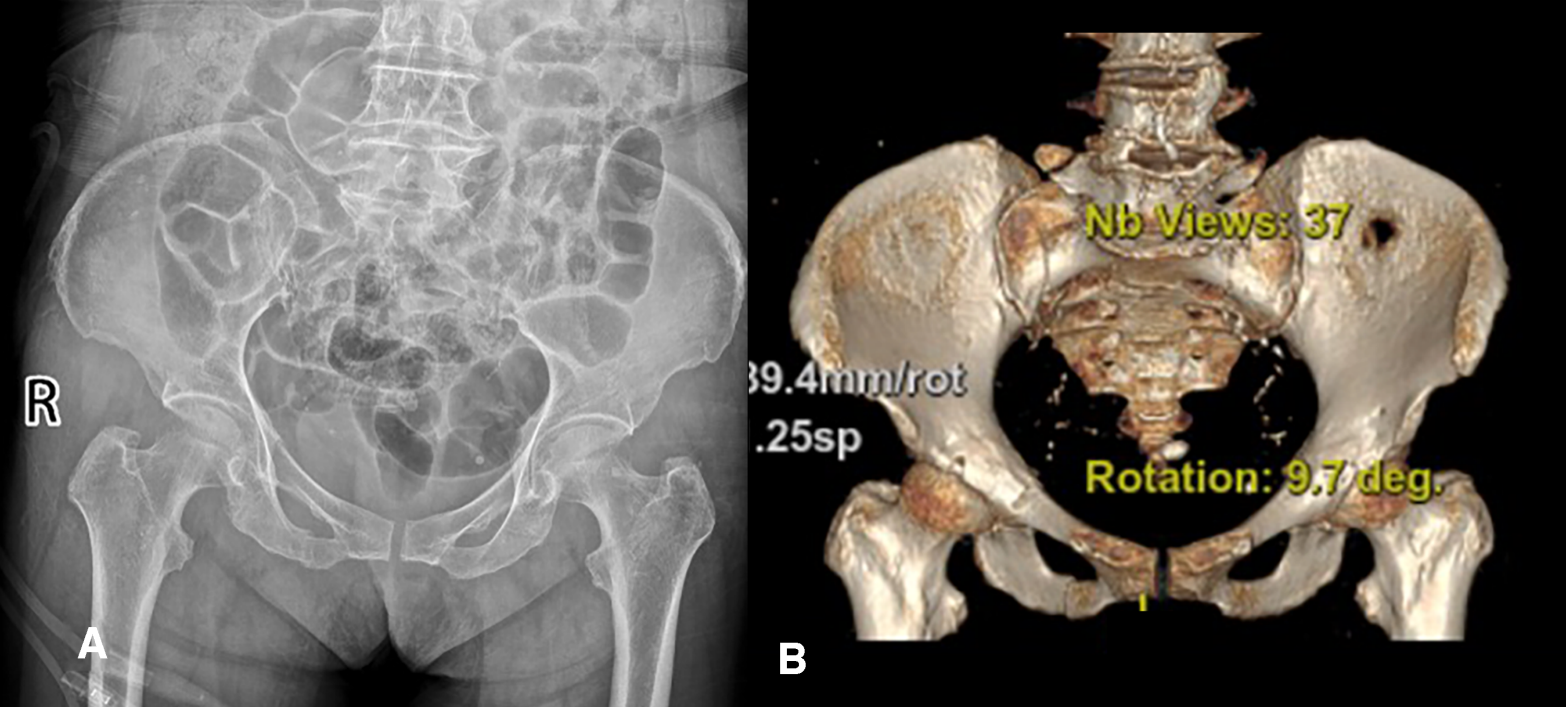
The imaging examination before surgery. (A) The anteroposterior radiograph of pelvis. (B) Three-dimensional reconstruction of pelvis.
